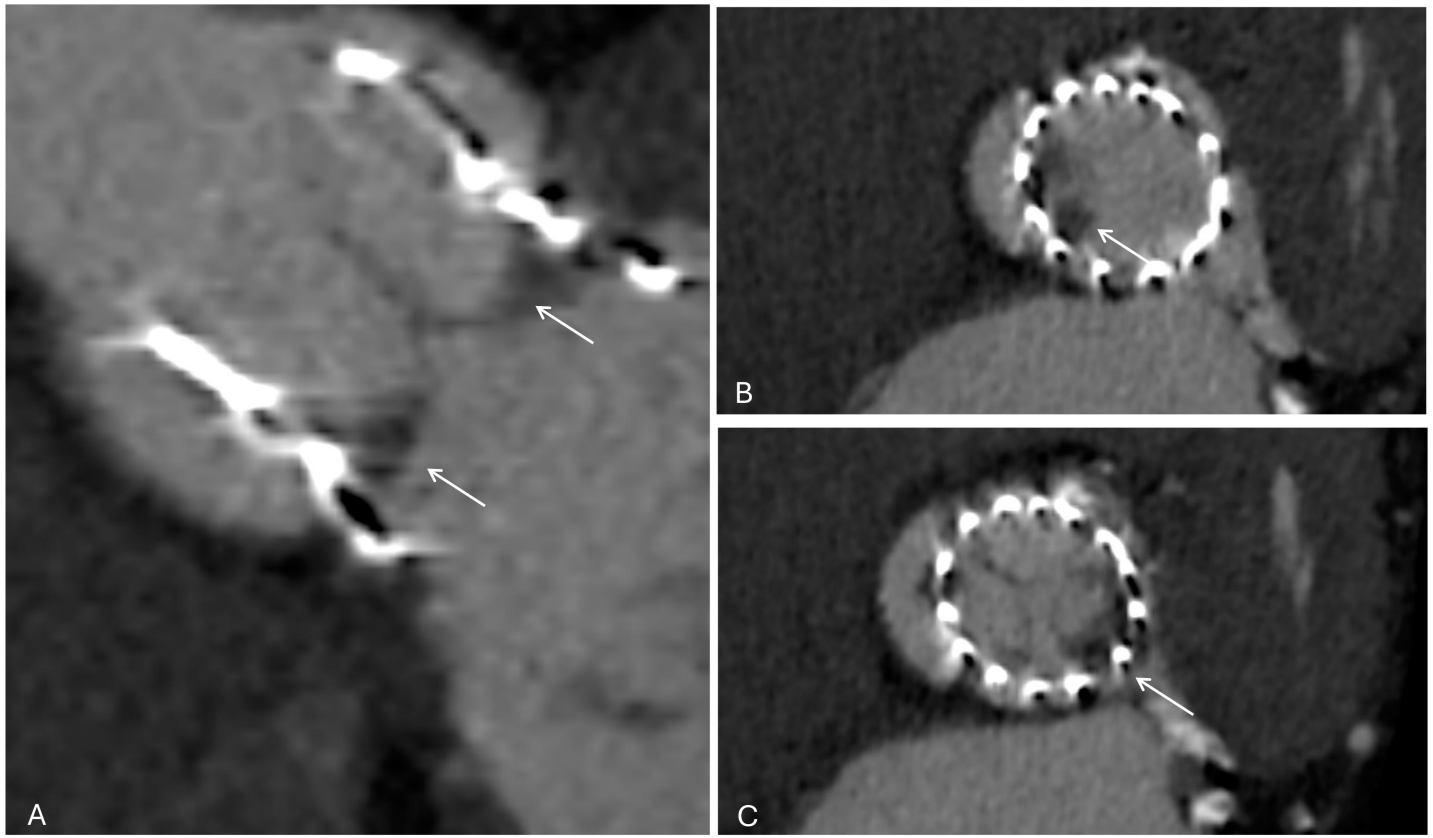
Cardiac CT demonstrating hypo-attenuated leaflet thickening
